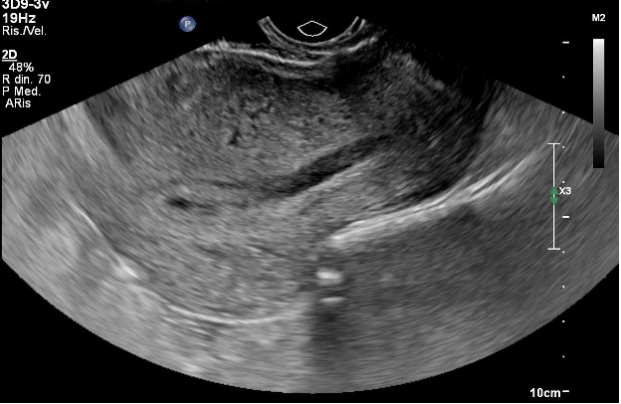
Ultrasound scan in the very early weeks of pregnancy, showing a thickened anterior wall with small anechogenic areas.
